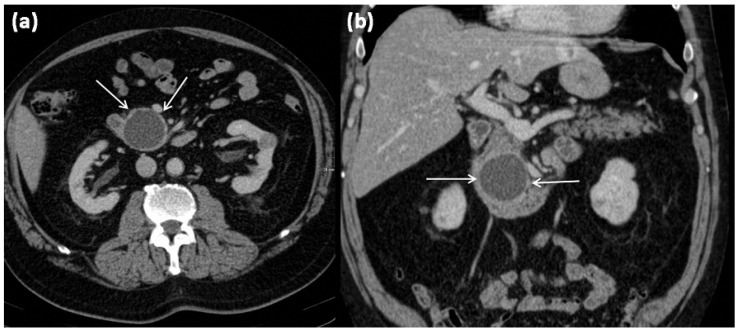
Axial (a) and coronal (b) contrast-enhanced computed tomography of the abdomen in venous phase in a 74-year-old male patient. In the processus uncinatus, a sharply demarcated pseudocyst (arrows) without solid components, septa, or surrounding infiltration can be seen. At most, the pseudocystic wall shows mild contrast enhancement.
